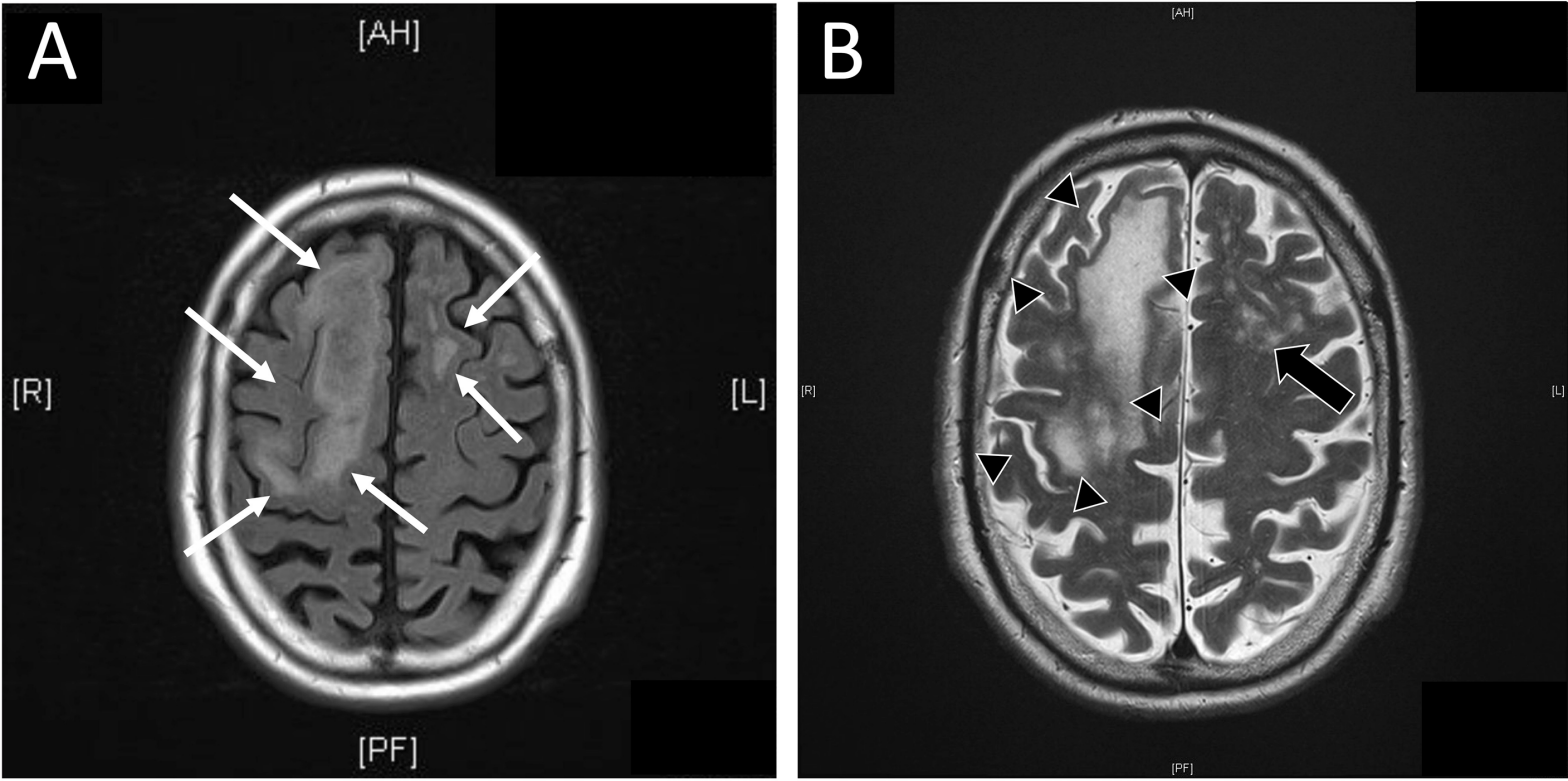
Magnetic resonance (MR) imaging of central nervous system. (A) Additional MR imaging was performed as advised after initial cranial computed tomography (cCT). In FLAIR weighted MR diffuse, subcortical located, edematous alterations of cerebral tissue were present (white arrows). (B) MR imaging showed subcortical located confluent (black arrow heads) or diffuse (black arrows) signal alterations in T2 weighted image in concordant locations reported in cCT. In opposite to CT, no hint for metastases was reported. Diffuse alterations of subcortical tissue are commonly seen in T2 or FLAIR weighted images in progressive multifocal leukoencephalopathy.
